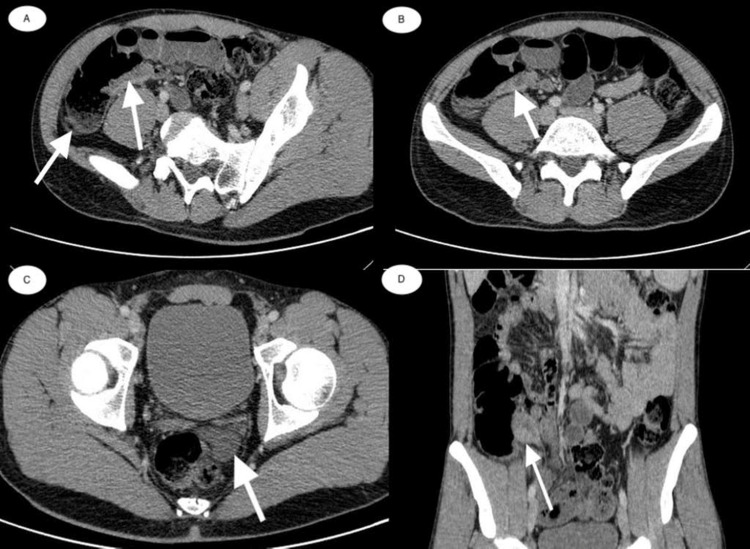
Contrast CT scans of the abdomen and pelvis A paracecal inflammatory area is noted in the right iliac fossa (A, B, and D) that is found all along the loop of the terminal ileum, measuring about 10 x 15 cm. It is determined by the highlighting of its wall as well as of the cecal wall. It is accompanied by intraperitoneal fluid in a small quantity that occupies the bottom of the Douglas sac and the perivesical sacs (C).
